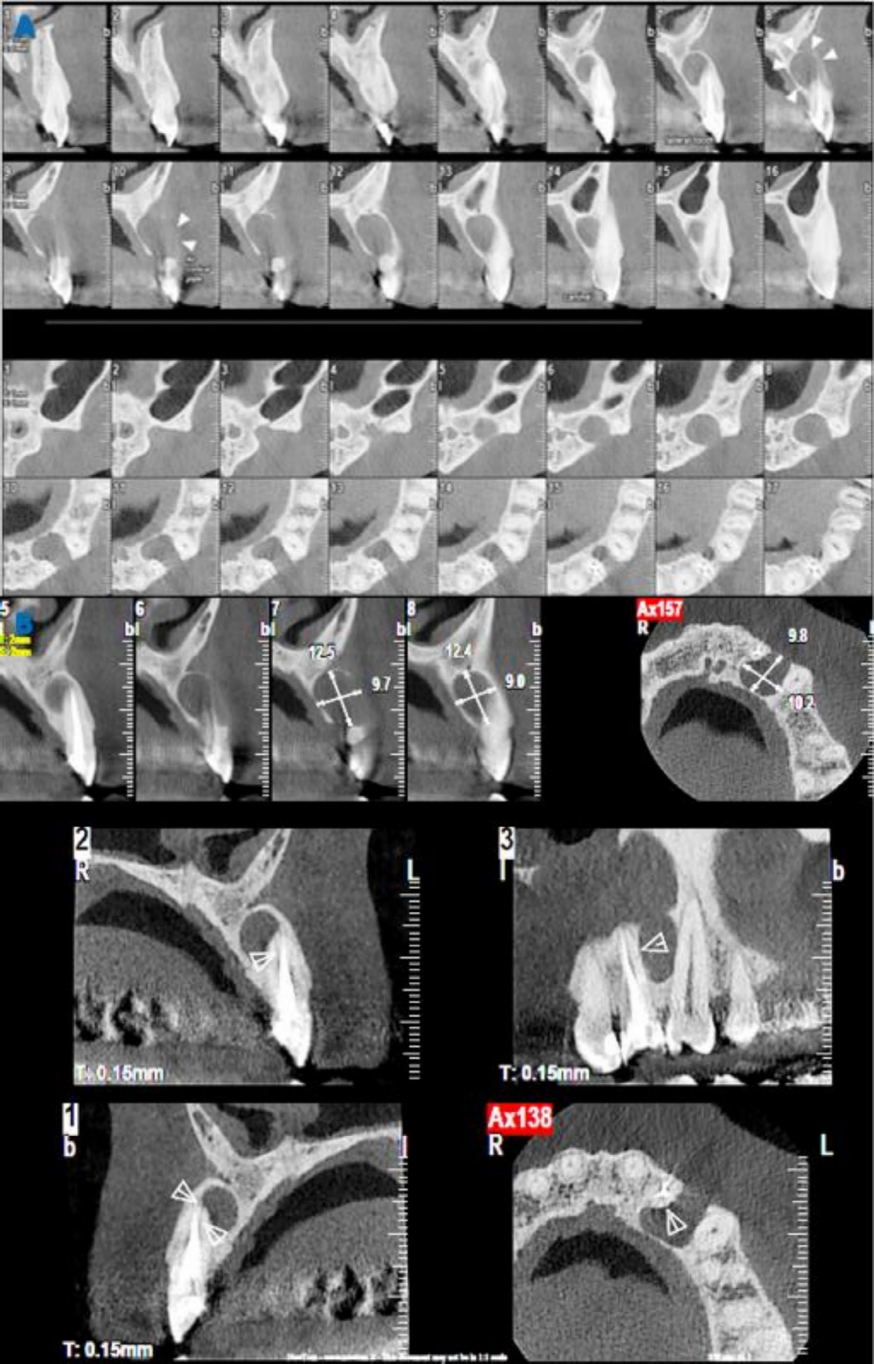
(A) CBCT images (axial and sagittal sections) indicating the extent of the lesion and associated buccal plate perforation; (B) Selected section of the possible fracture line.
